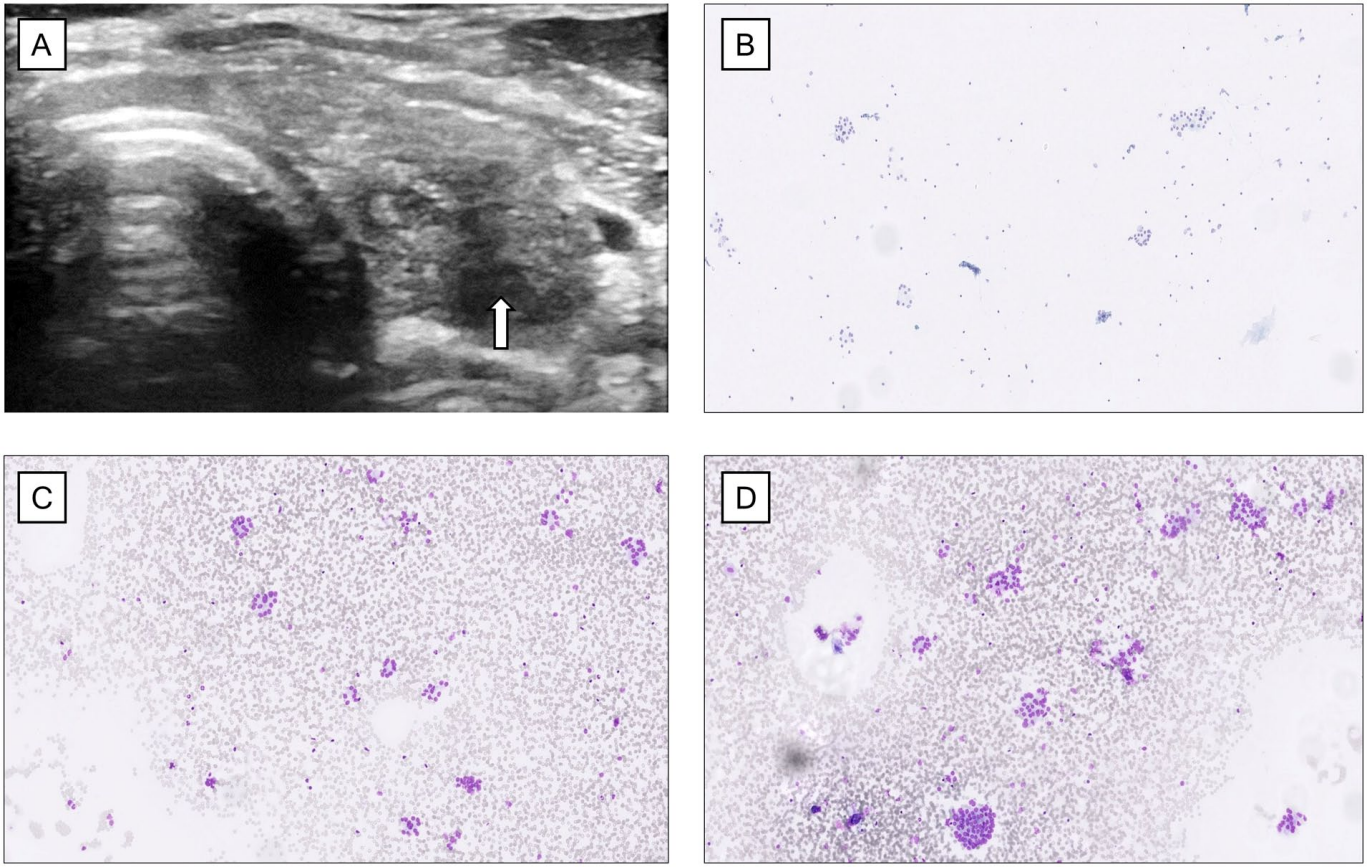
Ultrasound imaging of the left thyroid lobe revealed a well-demarcated area (white arrow) (a). Subsequent fine-needle aspiration cytology showed repetitive patterns of cells arranged in microfollicles, leading to a Bethesda IV category diagnosis (b–d).
